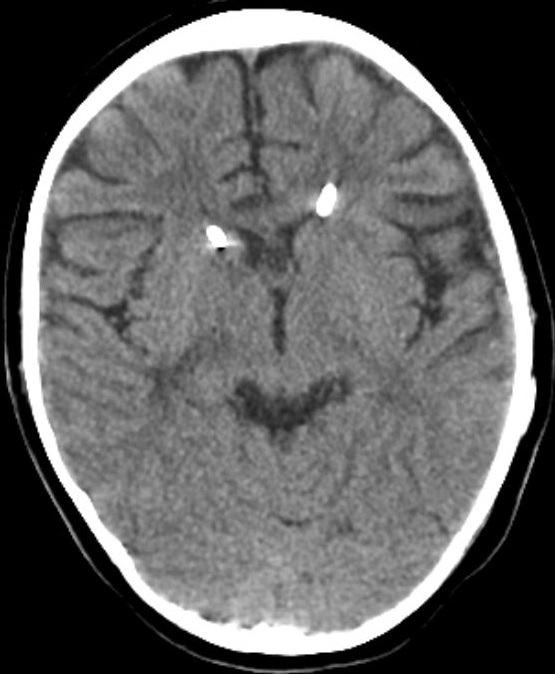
One year follow up CT scan showing the Cather sin place and the normal ventricular system
